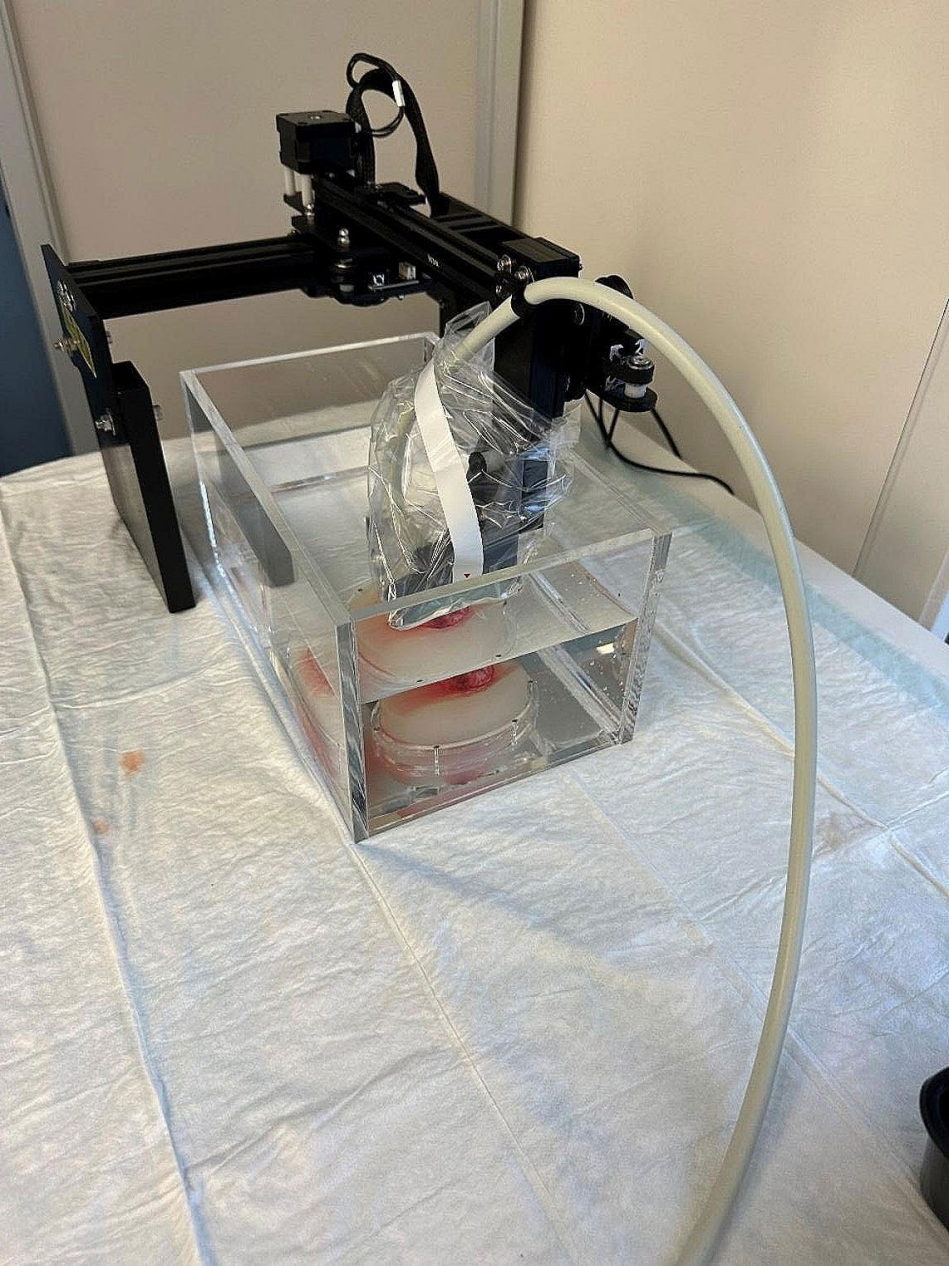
The motorized 3D ultrasound setup. The black system is the motorized rails. The transducer is placed below the saline surface so that the saline can transmit the sound waves [9]
